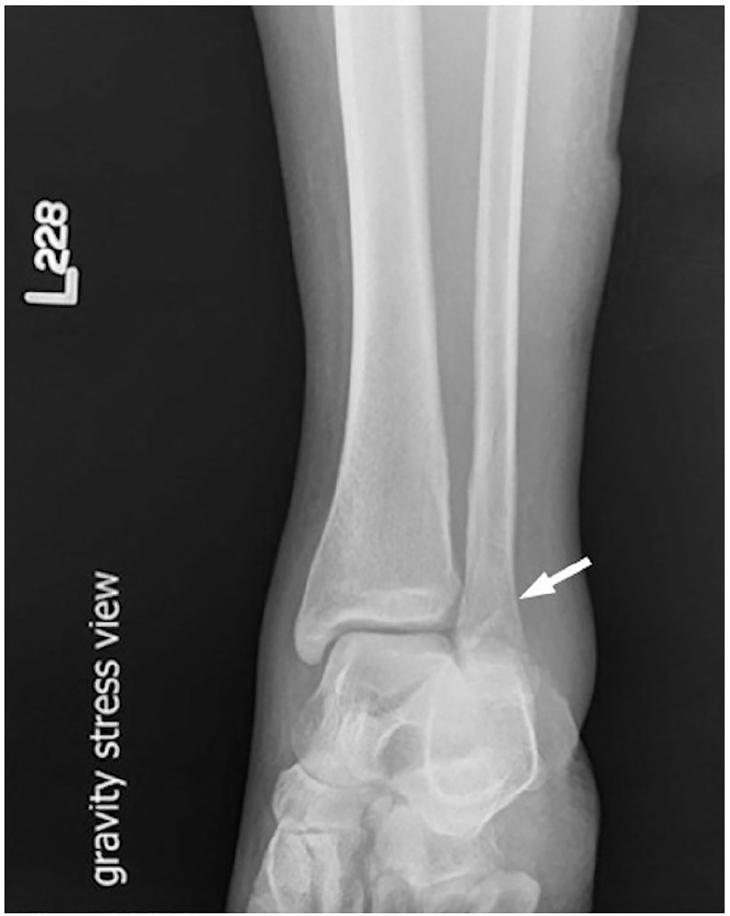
Stress view radiography of the ankle demonstrating an irregularity of the lateral malleolus cortex consistent with a non-displaced Weber B fracture.
